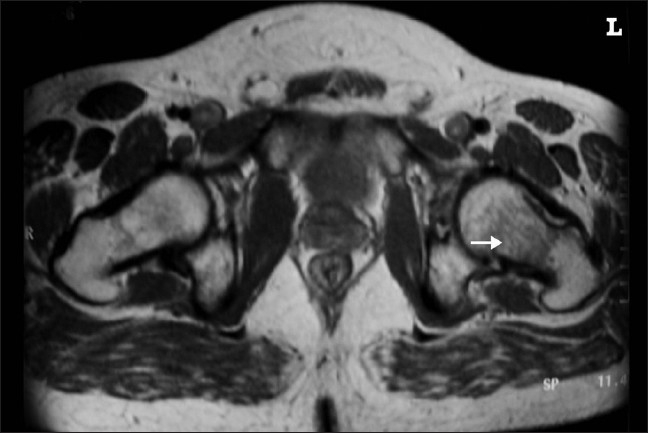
PD transverse T2-weighted image of left hip showing bone marrow edema (arrow)
